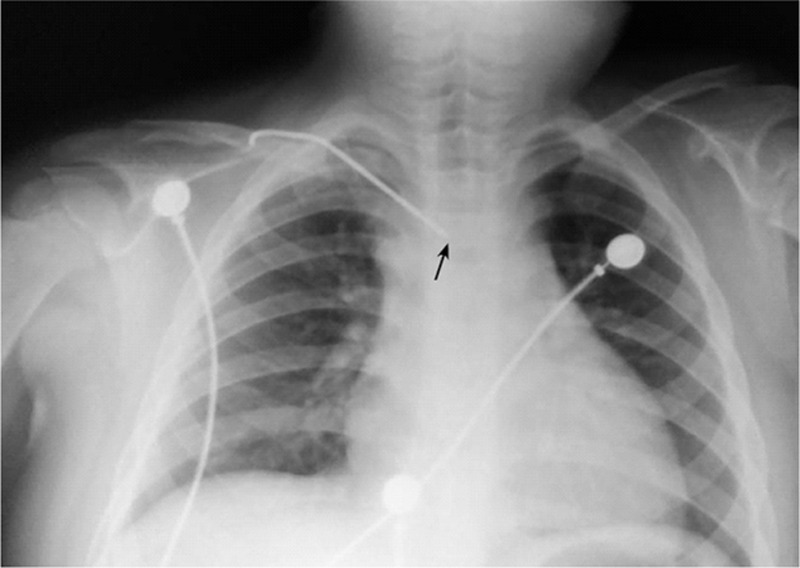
Chest x-ray showed migration of a bent Kirschner wire from the right clavicle. It was 9 cm in length, with its tip in the mediastinal shadow (black arrow).
